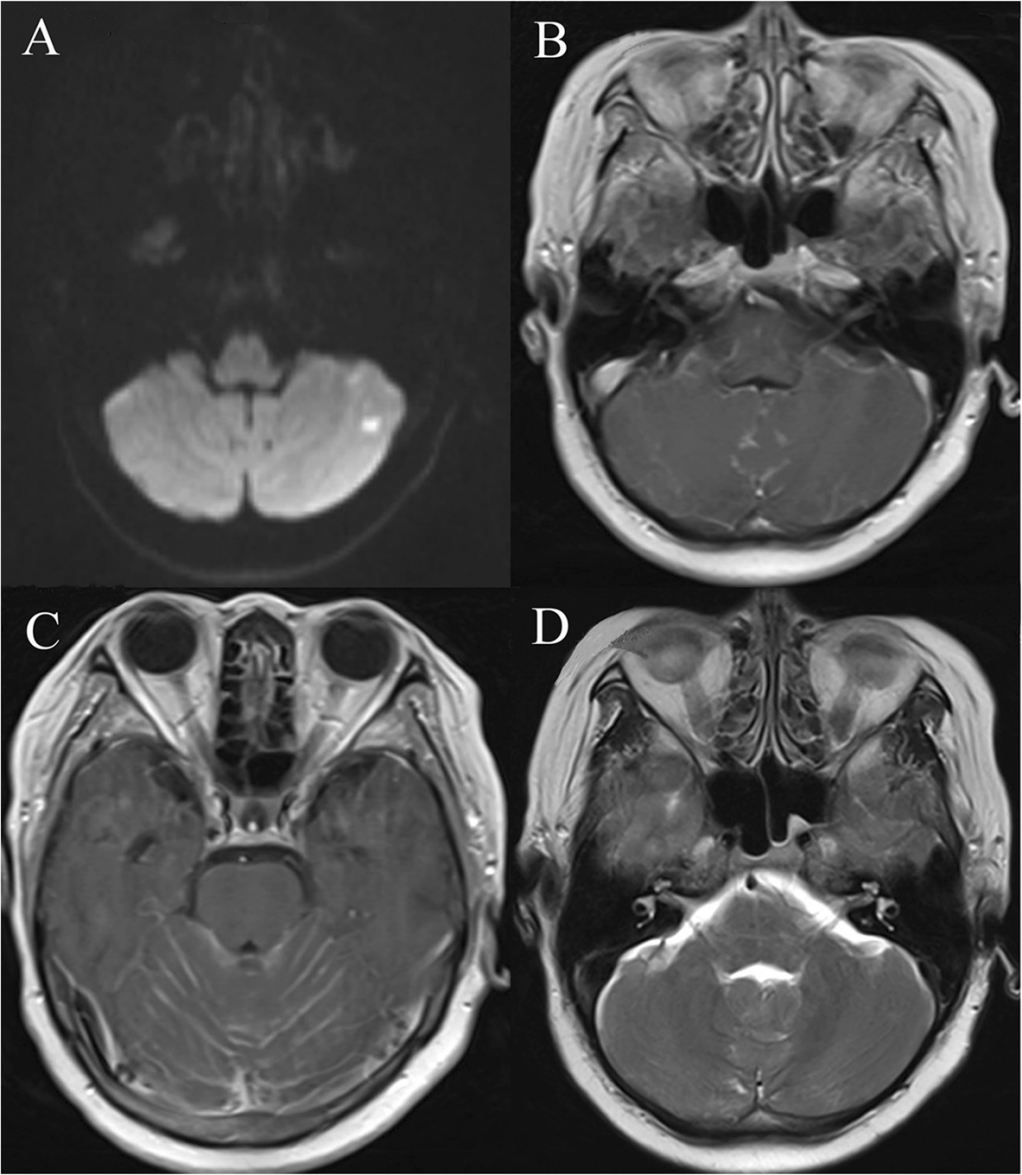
Magnetic resonance imaging (MRI) revealed the hot cross bun sign. (A) Diffusion-weighted MRI showing a patchy hyperintensity in the left cerebellar hemisphere; no enhancement was observed on contrast-enhanced MRI (B), suggesting cerebral infarction. (C) Axial T1-weighted MRI showing diffuse linear enhancement in the cerebellar sulcus, suggesting leptomeningeal metastases of breast cancer. (D) Axial T2-weighted MRI showing a cruciform hyperintensity in the pons; no atrophy was observed in the pons or cerebellum. All contrast-enhanced imaging was carried out with gadolinium-based contrast agents.
